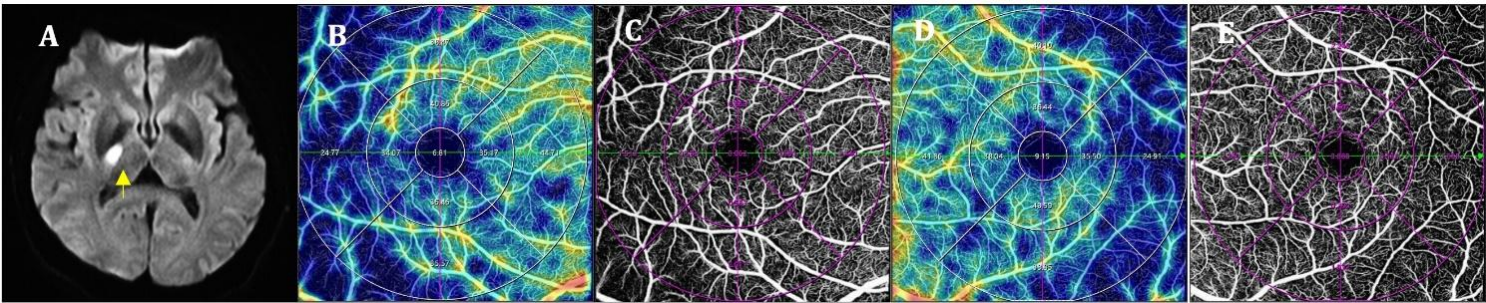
An example of OCTA findings in an ischemic stroke patient. A 50-year-old male was admitted to the hospital on February 1^st^, 2022, due to an infarction in the right internal capsule (yellow arrow, A). OCTA examination (one year after stroke onset) was conducted and revealed that the right eye exhibited a decrease in SRCP VD (B *vs.* D) and PA (C *vs.* E) compared with the left eye. MRI, magnetic resonance imaging; OCTA, optical coherence tomography angiography; SRCP, superficial retinal capillary plexus; VD, vessel density; PA, perfusion area.
